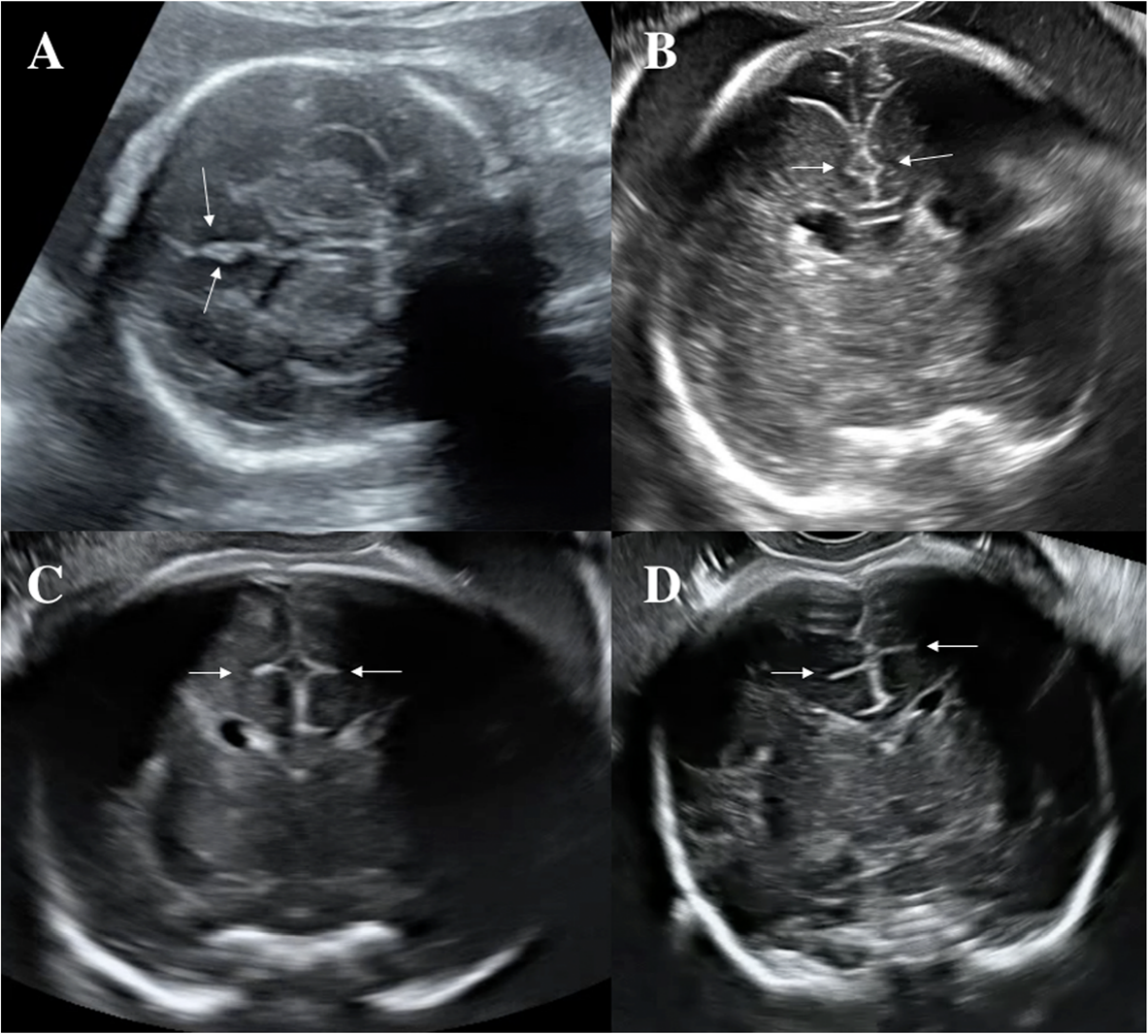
Asymmetry in the development and ‘catch-up growth’ of cingulate sulcus in a IMVM fetus with unilateral ventriculomegaly. Ultrasound image of cingulate sulcus on coronal transcaudate and transthalamic plane at 27 weeks (a), 30 weeks (b), 32 weeks (c) and 34 weeks (d) of gestation. a The cingulate sulcus was grade 0 on one side and was grade 1 on the other side. b The cingulate sulcus was grade 2 on one side and was grade 3 on the other side. c The cingulate sulcus was grade 3 on both sides. d The calcarine sulcus was grade 4 on both sides
